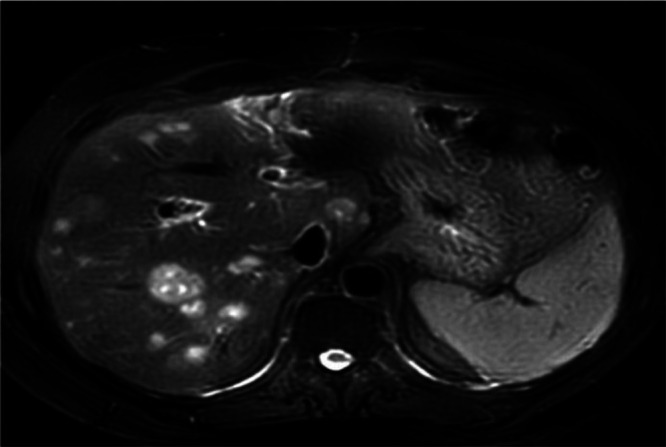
Computed tomography scans showing multiple hypoattenuation nodules.
